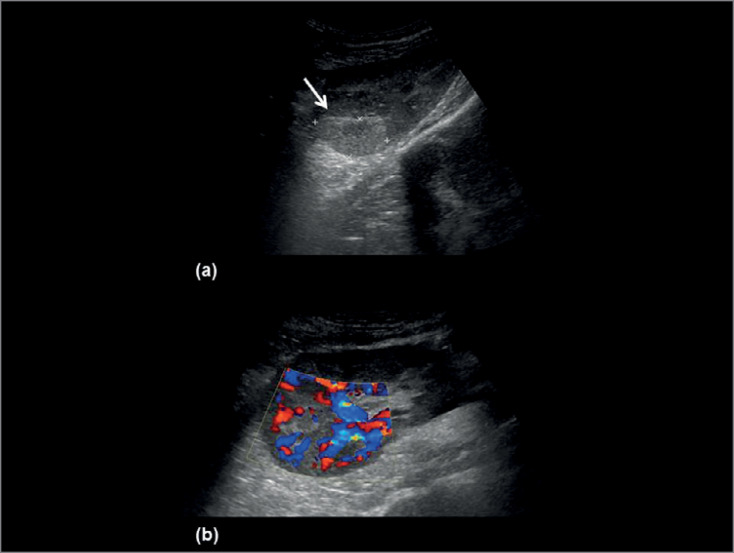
Panel A. Doppler ultrasound (DUS) showing rounded-shaped focal hyperechoic lesions (arrow); Panel B. Doppler ultrasound showing no perfusion abnormalities.
